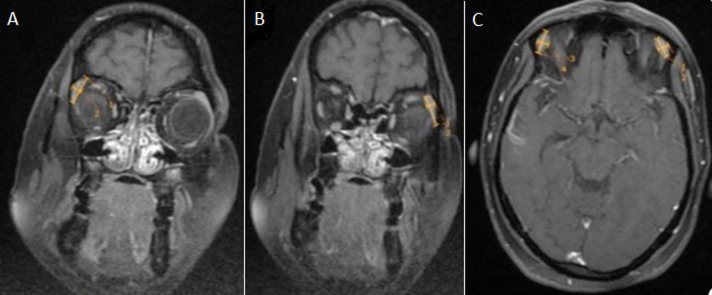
magnetic resonance imaging contrast-enhanced T1 weighted image of brain and orbit showing: A, B) coronal length and width of lacrimal gland on right and left sides; C) axial length and width of lacrimal gland on right and left sides
